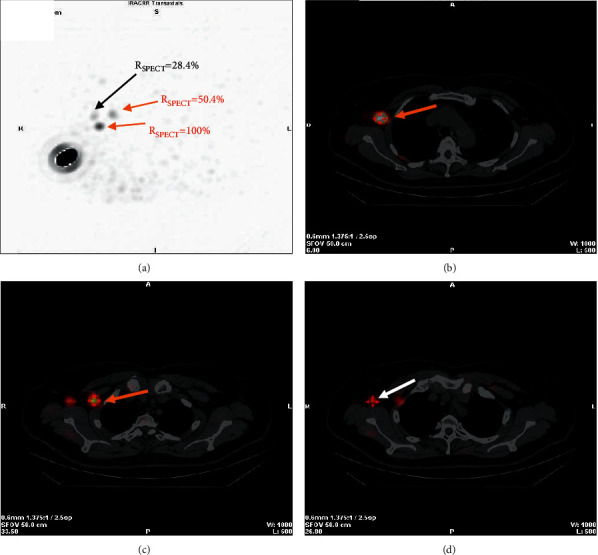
A 60-year-old female patient with invasive ductal carcinoma of the right breast. (a) Maximum intensity projection images. Three hot nodes are visualized with different *R*_SPECT_. Two nodes with higher *R*_SPECT_ (100%, 50.4%) are examined pathologically to be metastatic (red arrows), and another node with lower *R*_SPECT_ (28.4%) is healthy (black arrow). (b–d), SPECT/CT hybrid images for localizing the two metastatic nodes (red arrows) and the healthy node (white arrow). The surgeon might selectively avoid excising lymph nodes with lower radioactivity (*R*_SPECT_ ≤ 30%) deriving from preoperative SPECT/CT images.
